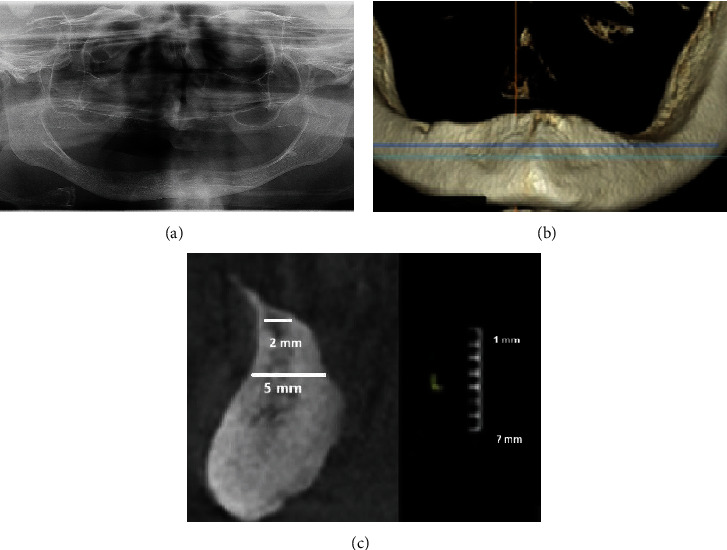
Preoperative radiological images: (a) panoramic radiogram, (b) CBCT shows a pointed top of the alveolar ridge, and (c) a sagittal view of the mandible with a gauge presenting the amount of leveling (osteotomy) that would be necessary to obtain the 5-mm width.
